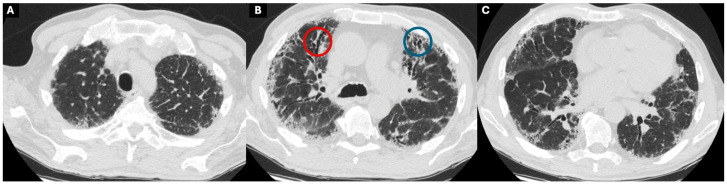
Chest CT images of a patient with fibrosis-predominant ILD showing honeycombing (image (B)), traction bronchiectasis (image (B)), and interstitial thickening. Image (A–C) show cross-sectional views of chest CT images of a patient with fibrosis-predominant ILD. Red circle shows traction bronchiectasis and blue circle shows honeycombing.
